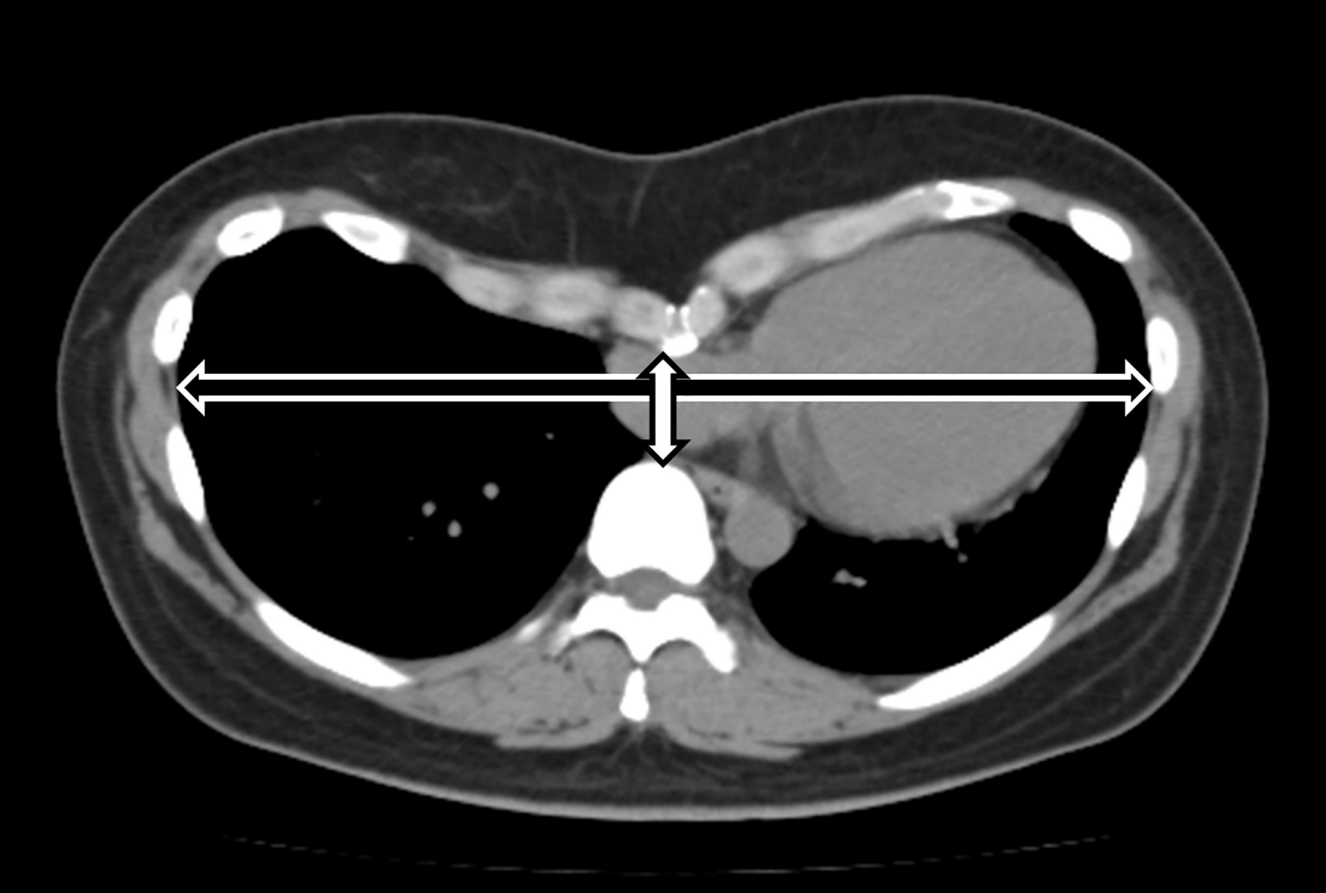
Measurement of the Haller computed tomography (CT) index. The Haller CT index was calculated by dividing the thoracic transverse diameter (black bidirectional arrow) by the distance between the sternum and the vertebra (white bidirectional arrow).
